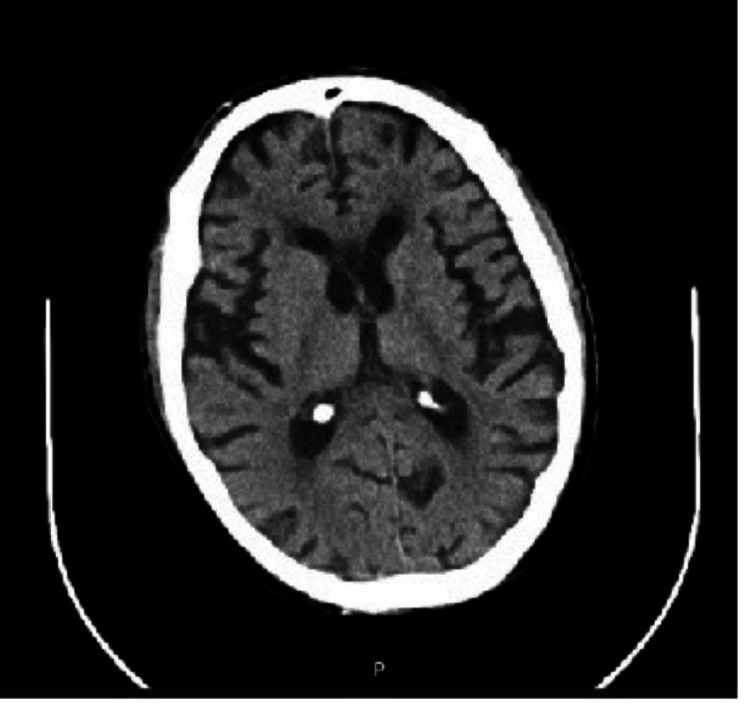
Cranial computed tomography showing generalized parenchymal atrophy and mild periventricular leukoencephalopathy
